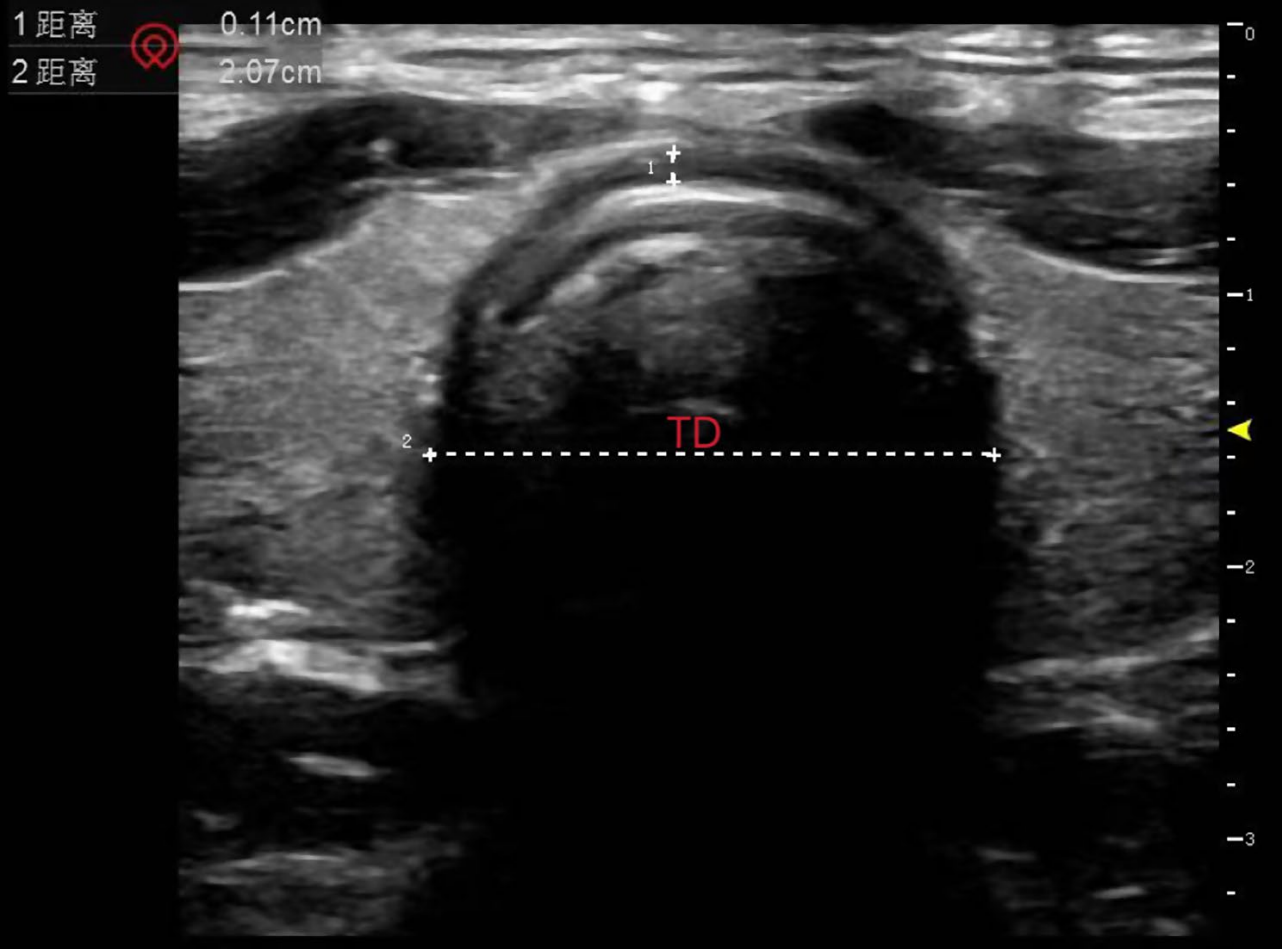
The TD at the level of the suprasternal notch measured by ultrasound. The high-frequency hockey stick probe was coated with ultrasound gel before being held perpendicular to the midline of the trachea and the TD at the level of the suprasternal notch was measured. The shadow: trachea; TD: tracheal transverse diameter
